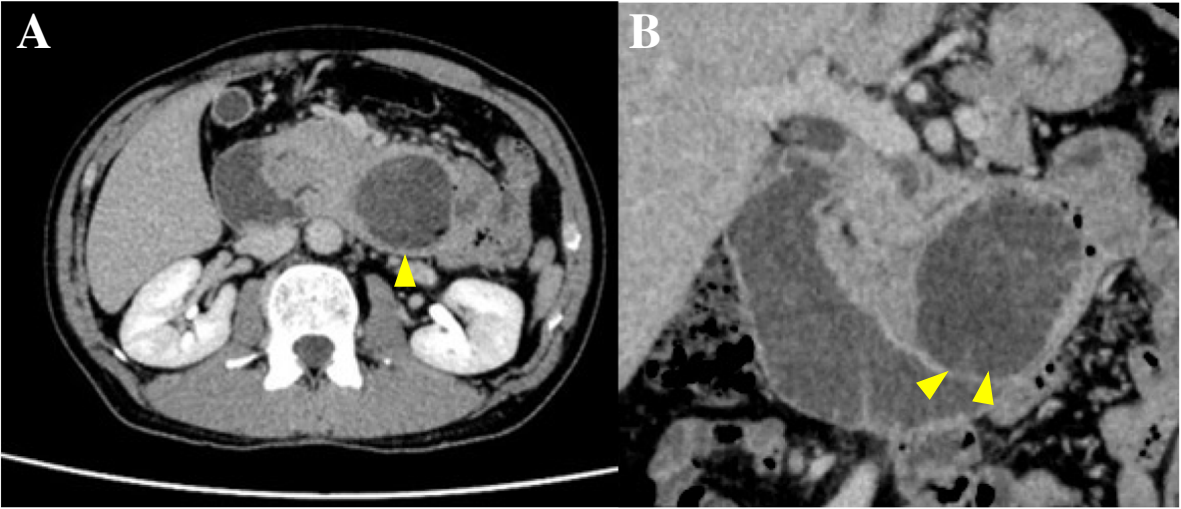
Abdominal CT showed 6 cm low-density mass in the retroperitoneum, in all phase (arrow). a Coronal view showed gastroduodenal invagination. b Duodenum proximal to the mass is dilatated (arrow)
